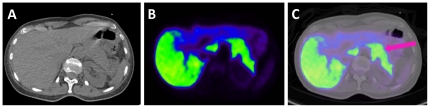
PET-CT of abdomen at approximately 30 minutes after injection of ^11^C-CUMI-101 (369 MBq) shows high uptake in pancreas. A: Axial CT of abdomen, with right side of subject on left, and anterior aspect facing upward on page. B: PET image of same cross-section, showing high uptake of ^11^C-CUMI-101 in pancreas and liver. C: Fused PET-CT image confirms that radioactive signal on the left is at anatomical location of pancreas (pink arrow).
